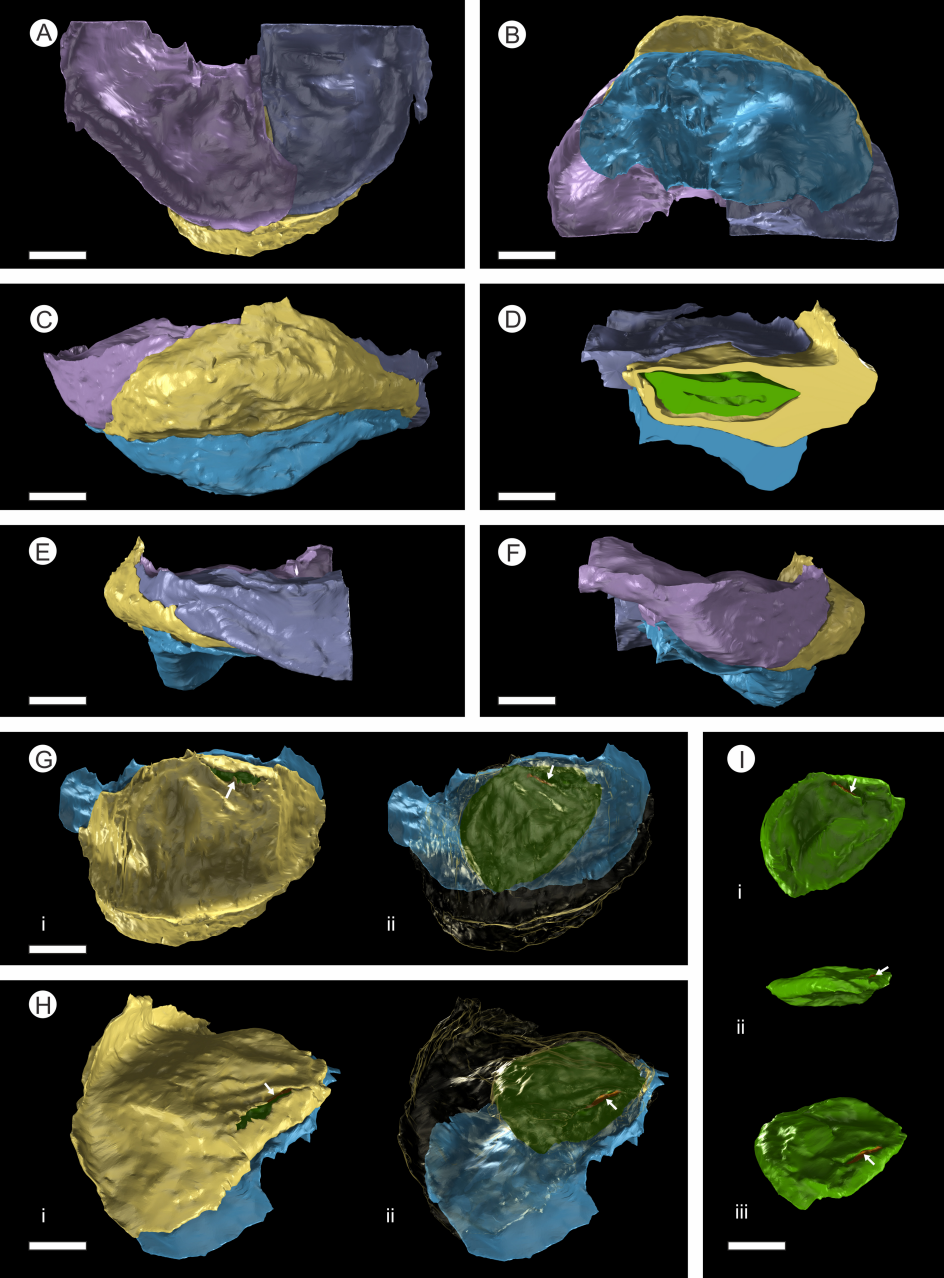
3D Model from DLS synchrotron x-ray dataset showing high resolution reconstruction of a bract/scale complex constrained by overlying bracts. (A) Top view of bract/scale complex; (B) Basal view of bract/scale complex; (C) Frontal view of bract/scale complex; (D) Virtual slice through bract/scale complexes showing internal morphological relationships; (E) Right-hand side view of bract/scale complex; (F) Left-hand side view of bract/scale complex; (G) Top view of bract/scale complex with overlying bracts removed (i), ovule position and micropylar region (see arrow) seen through transparent scale (ii); (H) Tilted side view of bract/scale complex with overlying bracts removed (i), ovule position and micropylar region (see arrow) seen through transparent scale (ii); (I) View of ovule and position of micropylar region (see arrow) seen in three views: (i) top, (ii) side, and (iii) tilted side. All Scale Bars = 1 mm. Key: blue, bract of bract/scale complex; yellow, ovuliferous scale of bract/scale complex; purple/mauve, overlaying bracts; green, ovule; orange, micropylar region.
